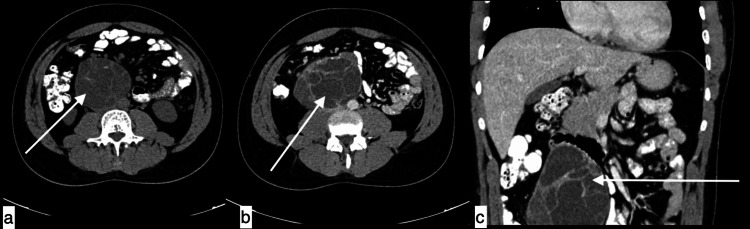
Retroperitoneal hydatid cyst A 26-year-old male with a retroperitoneal hydatid cyst presented with right flank pain and discomfort. Axial plain (a), axial post-contrast (b) and reformatted coronal post-contrast (c) CT showing a large well defined heterogeneously hypodense solid cystic multiloculated lesion in retroperitoneum with enhancing walls and internal septations with non-enhancing hypodense exophytic component, displacing bowel loops anteriorly, abutting and compressing IVC and aorta posteriorly with contact area >180 degrees. IVC: inferior vena cava
